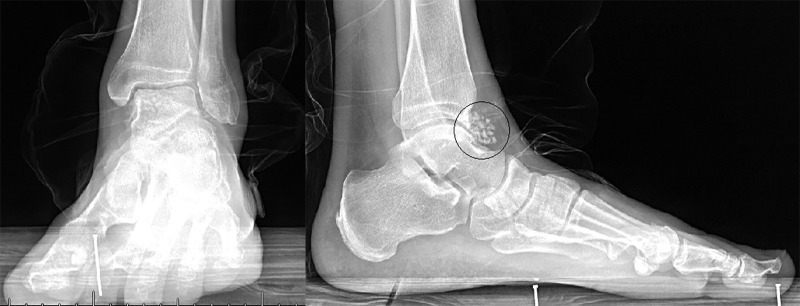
Preoperative Radiographs. Preoperative radiographs demonstrate innumerable subcentimeter loose bodies within the tibiotalar joint. Anteroposterior radiograph shown on the left, and lateral radiograph shown on the right with loose bodies outlined by black circle.
